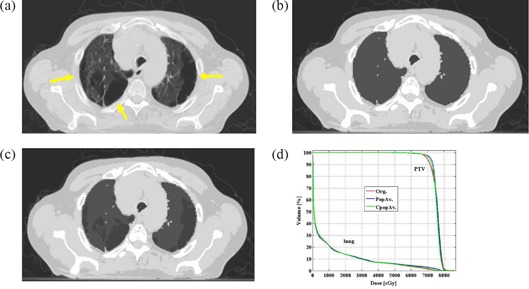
Example of a patient with bullous formations (yellow arrows) in both lungs. original treatment planning CT image (lung window); (b) treatment planning CT image of the same slice as with the 5 bulk densities applied; (c) treatment planning CT image of the same slice as and where air density is applied to the bullous formations of both lungs; (d) DVH comparing the PTV and lung of the 3 plans.
